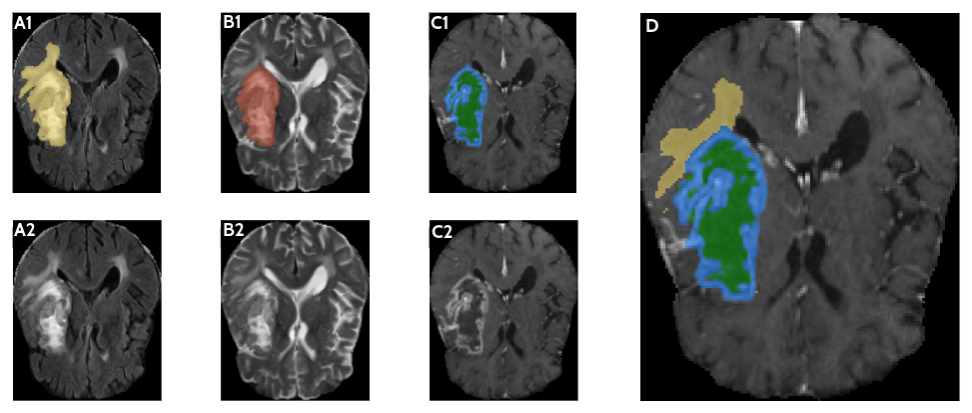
Tumor regions with MRI modalities ((A1) = WT overlaid on the FLAIR MRI slice, (A2) = FLAIR MRI slice, (B1) = TC overlaid on the T2 MRI slice, (B2) = T2 MRI slice, (C1) = ET overlaid on the T1CE MRI slice, (C2) = T1CE MRI slice) and their combination (D). From left to right: WT (yellow), TC (red), ET (blue), necrotic structure (green).
